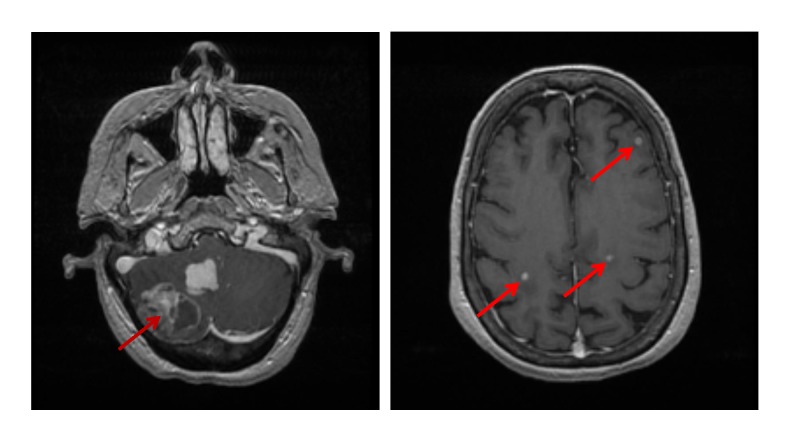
MRI scans of the brain of the patient at initial presentation Brain metastases are highlighted by arrows. Two large metastases are seen in the cerebellum (left image). Additional small metastases are diffusely distributed (right image). The patient subsequently had whole brain radiation.
